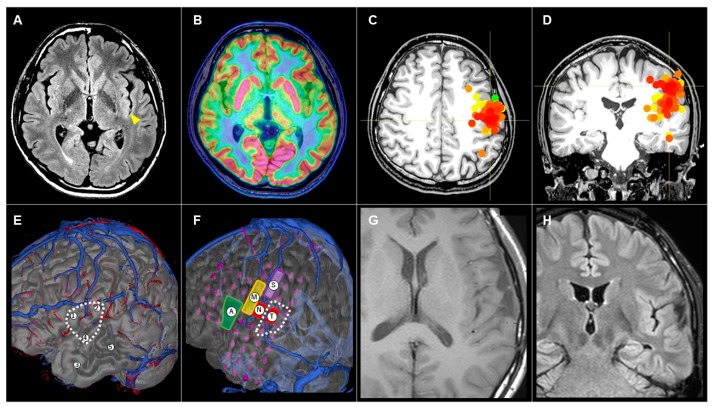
(A) Preoperative axial magnetic resonance imaging (MRI) showing a slight signal change in the posterior part of the left insula (yellow arrowhead). (B) No areas of low uptake are evident on axial 18F-fluorodeoxyglucose positron emission tomography. (C,D) Estimated dipoles in magnetoencephalography are demonstrated by red and orange dots on the axial (C) and coronal (D) images. (E) Lateral view of 3D reconstruction MRI. Locations of five SEEG electrodes and the suspected area of epileptogenicity were demonstrated. (F) Lateral view of 3D reconstruction MRI. Locations of SDG electrodes, area of induced symptoms, and area of resection, showing hand motor symptoms (M), hand sensory symptoms (S), negative motor symptoms (N), anomia (A), and tongue sensory symptoms (T). (G,H) Postoperative axial (G) and coronal (H) MRI showing partial removal of the superior temporal gyrus.
